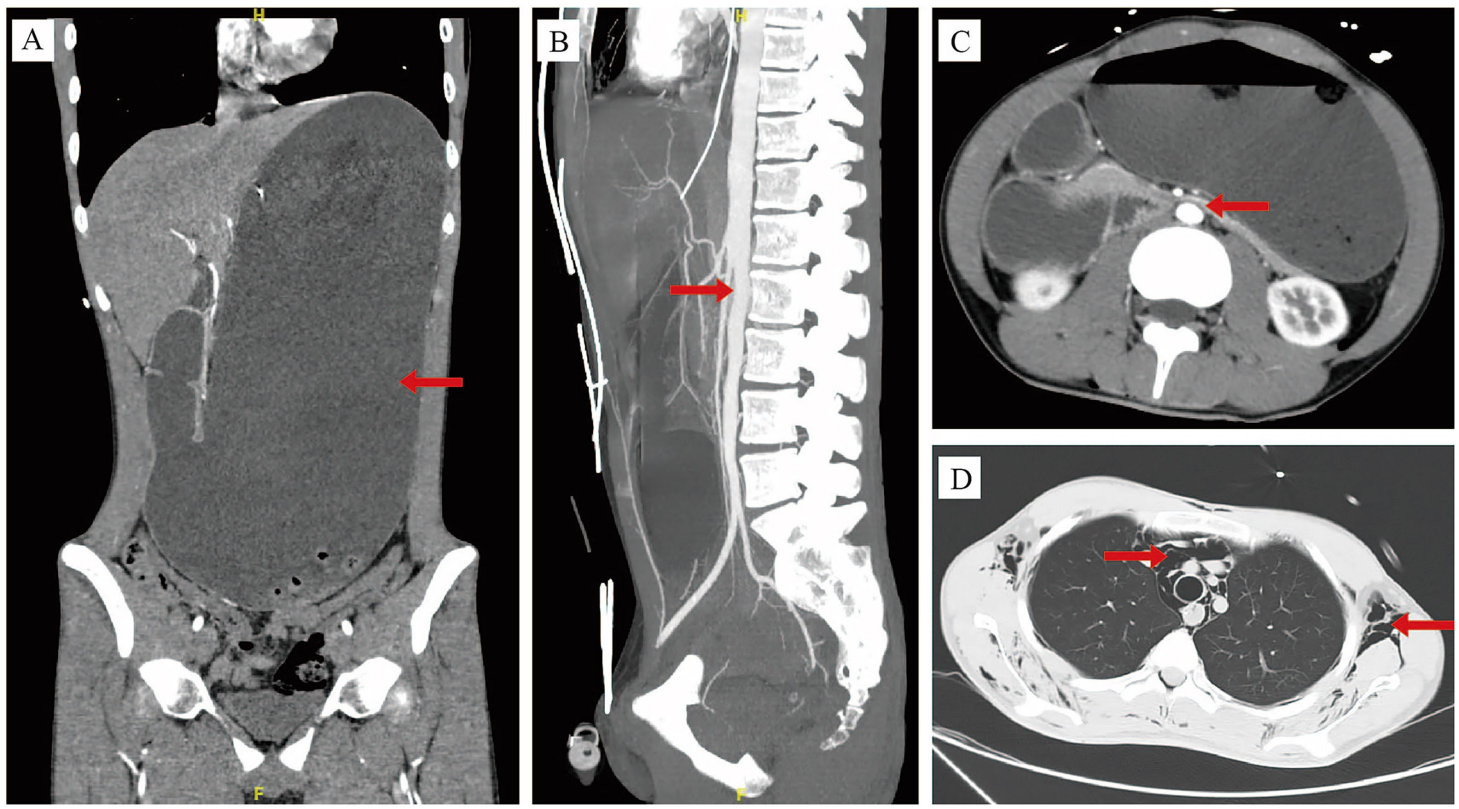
(A) Contrast-enhanced computed tomography showed massive gastric dilation. (B) The angle between the superior mesenteric artery and the abdominal aorta was approximately 15°. (C) Duodenal obstruction due to vascular compression. (D) Subcutaneous emphysema and pneumomediastinum.
